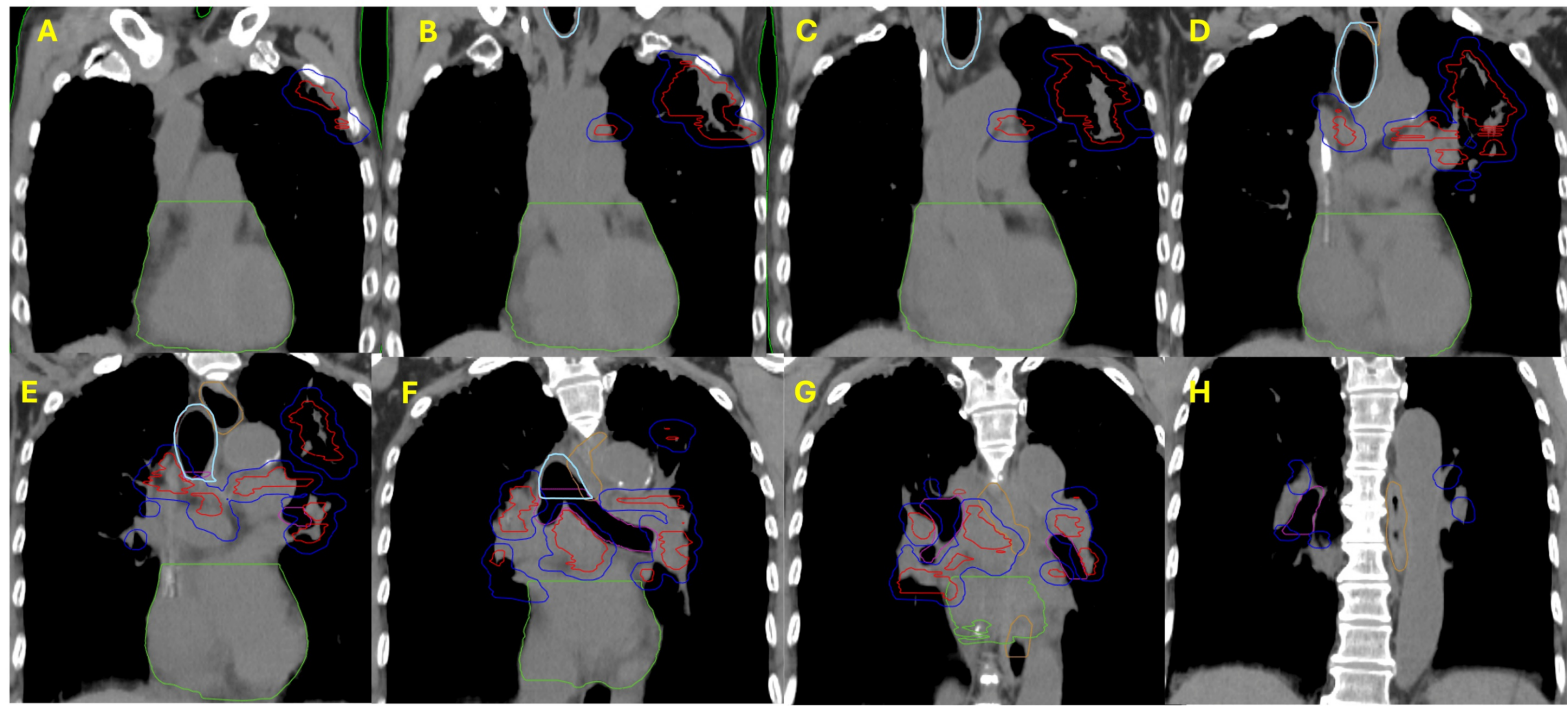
Planning CT contours of the left lung and mediastinal non-small cell lung cancer (NSCLC) Coronal planning CT slices with contours display gross tumor volume (red), planning target volume (blue), heart (green), trachea and large bronchus (light blue), esophagus (orange), and bronchus/small airway (pink). Slices are shown every 1 cm and go from the anterior to the posterior from the (A) anteriormost slice, showing the anterior extent of the disease, which extends towards the second and third ribs in the left lung. Additional posterior slices (B, C) reveal bulky left lung and left mediastinal disease, and slices (D-G) demonstrate the disease extending to the right mediastinum. The most posterior CT slice (H) depicts the posterior extent of the planning target volume, with no additional gross tumor volume.
